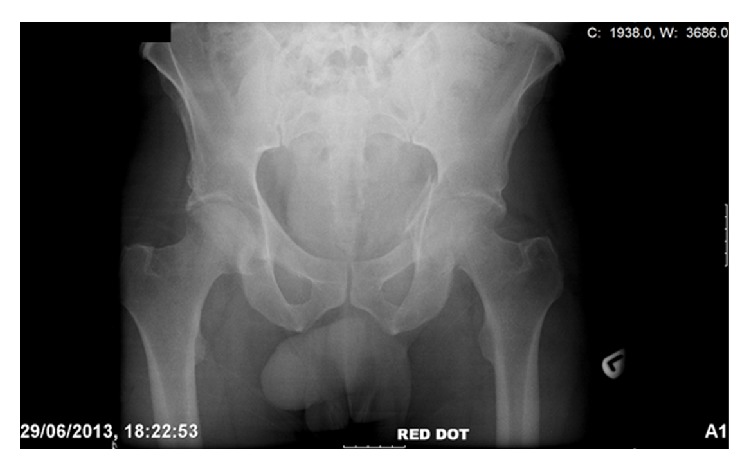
An AP radiograph taken at the time of initial presentation revealing a left acetabular fracture.
